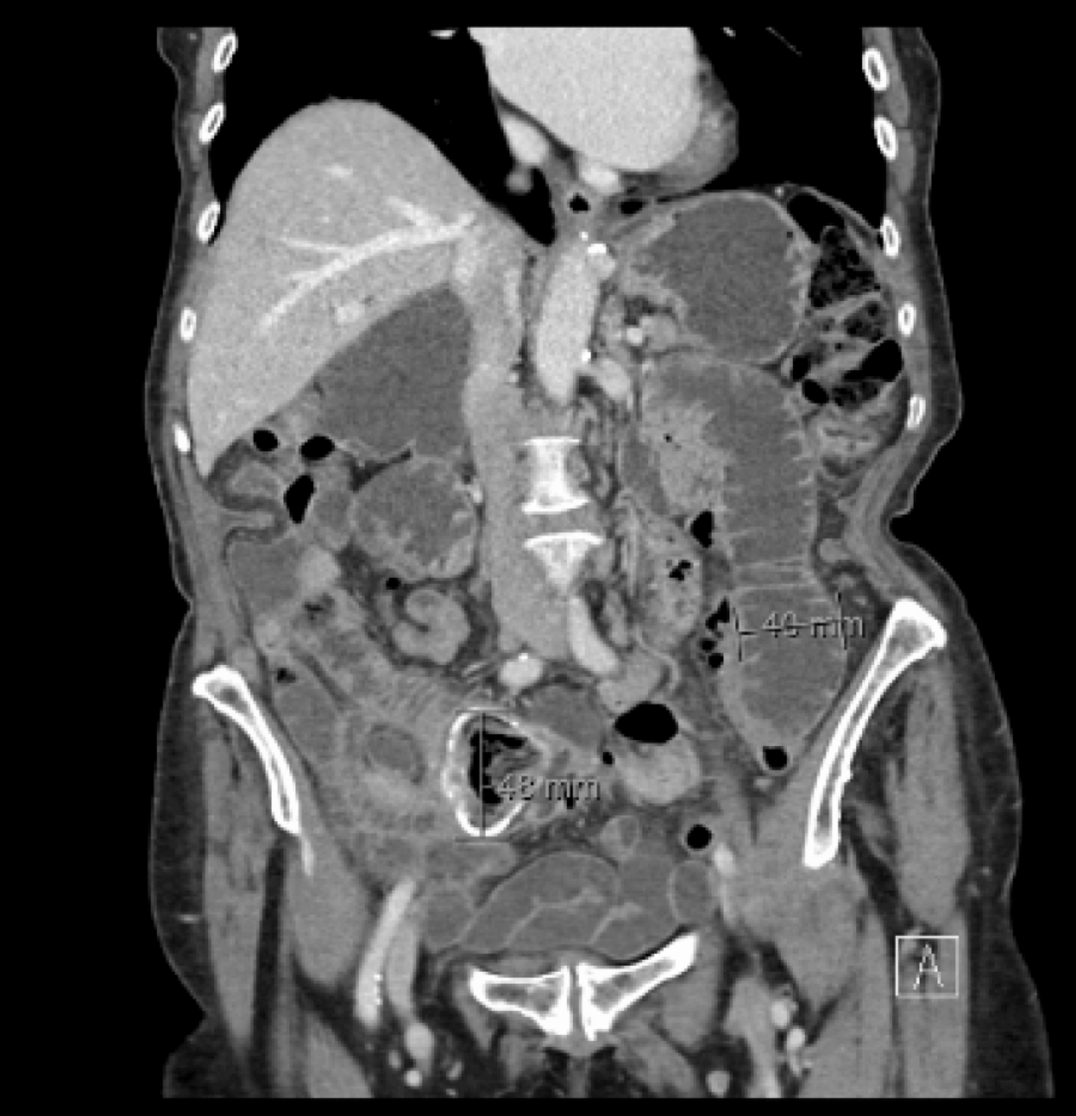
Coronal CT Coronal CT showing severely distended bowel loops and a heterogeneous structure with peripheral curvilinear calcification measuring up to 48 mm.
